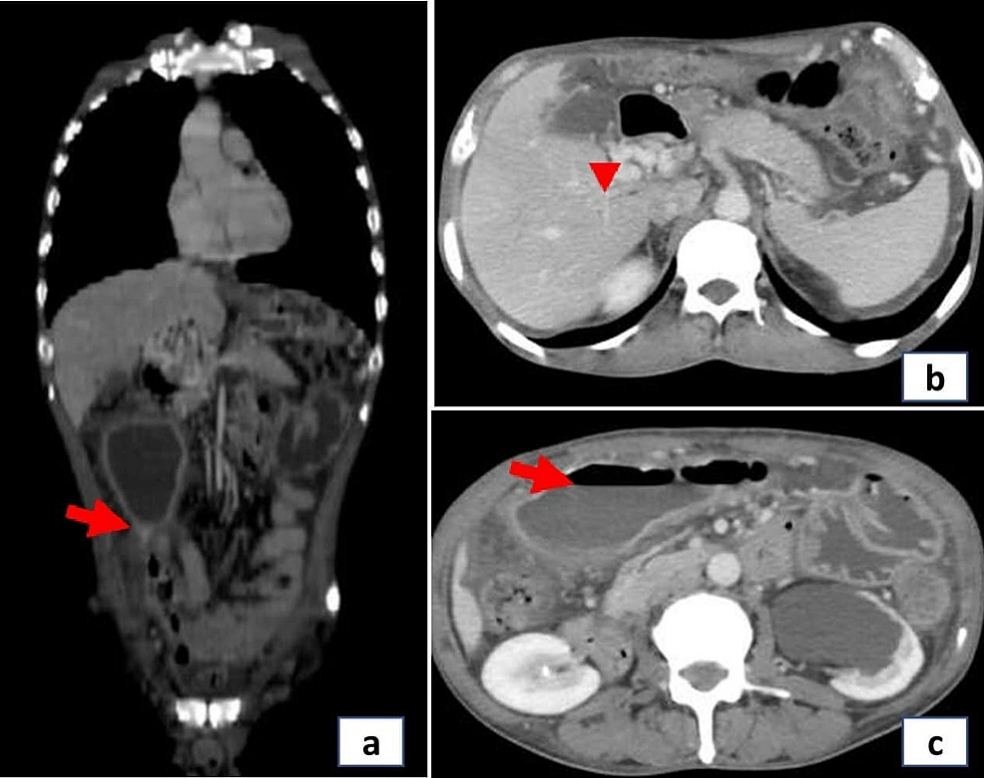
Contrast-enhanced CT abdomen images: a) arrow pointing to a stricture in proximal jejunum, b) arrow head pointing multiple collaterals in the portomesenteric confluence and superior mesenteric vein, c) arrow pointing to upstream dilatation of the bowel with air fluid levels
